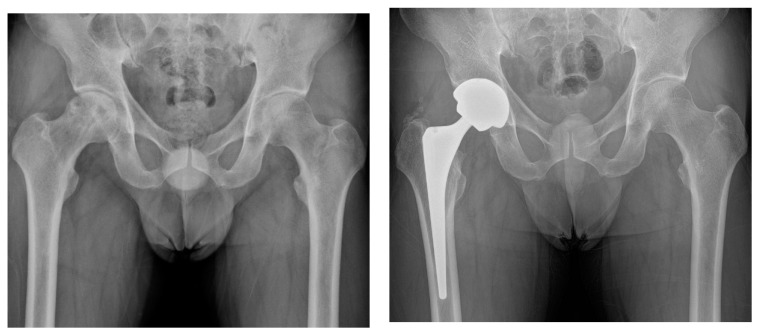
(A) Preoperative hip radiograph of a 41-year-old man who had undergone surgical removal of meningioma in the right frontal lobe 4 years prior to THA, demonstrating collapsed osteonecrosis of the right femoral head. (B) Five-year postoperative radiograph demonstrating stable implants with Brooker grade 2 heterotopic ossification. The patient had no pain and was satisfied with the outcome.
